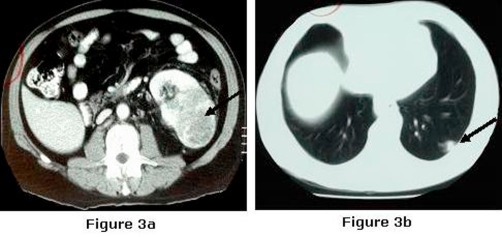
(A) Computed tomography of abdomen and thorax. showing left sided renal cell carcinoma, (B) Computed tomography of abdomen and thorax Left sub-pleural lesion suspicious of metastasis.
